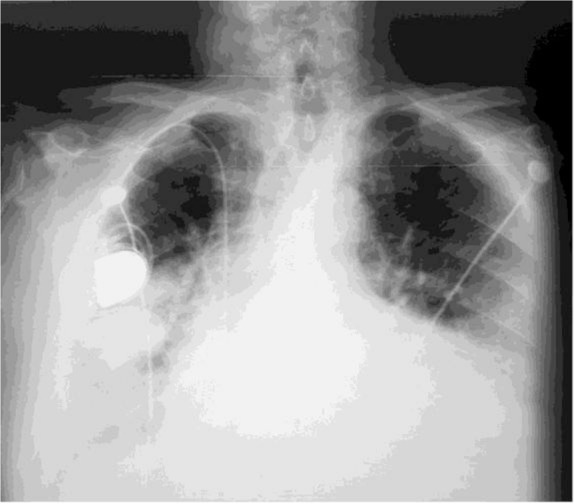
Chest X-ray showing three mass-like opacities in the right lung
